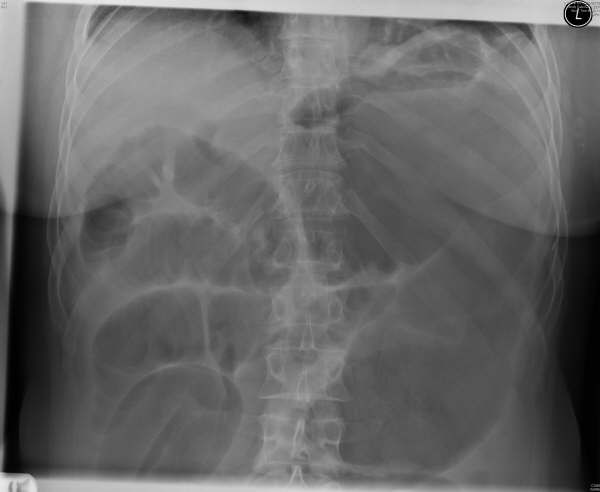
Plain abdominal x-ray showing small bowel obstruction with dilated large bowel in the left upper quadrant.
